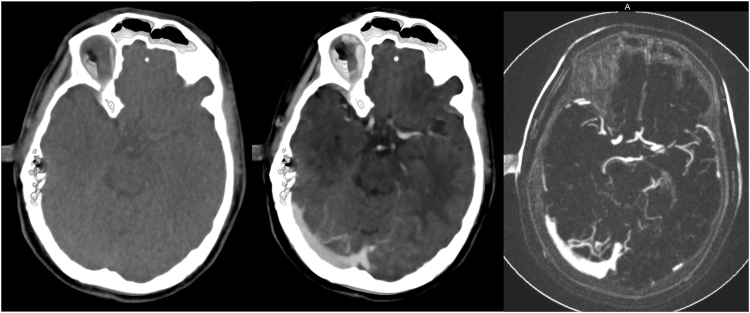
Left to right: First time point of 4D CT acquisition, WTA, and WTV. The WTV is highly sensitive to contrast changes over time making it a promising feature for vessel segmentation.
